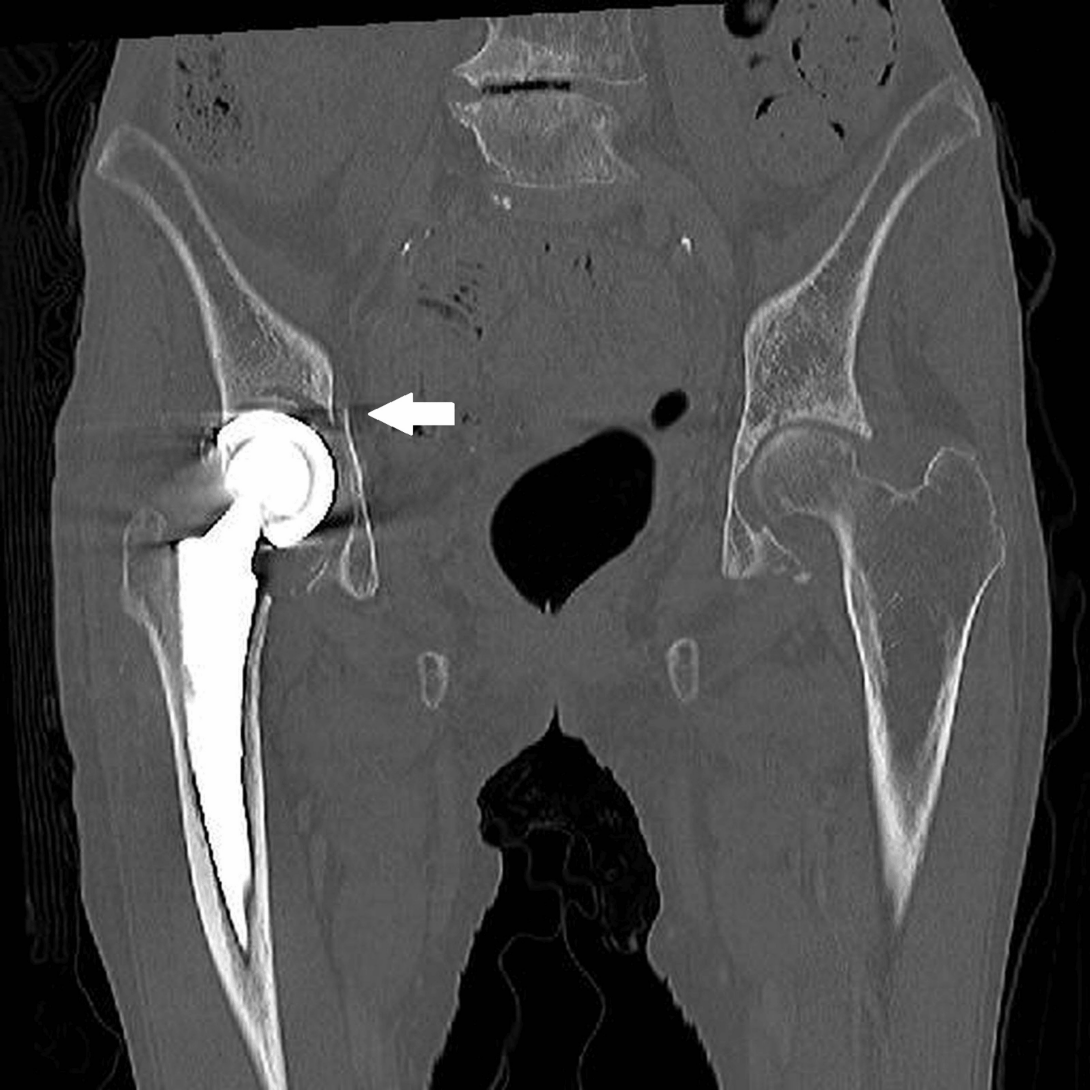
Pelvic CT coronal image on admission. A right acetabular fracture was identified (arrow), with no obvious loosening of the femoral stem.
